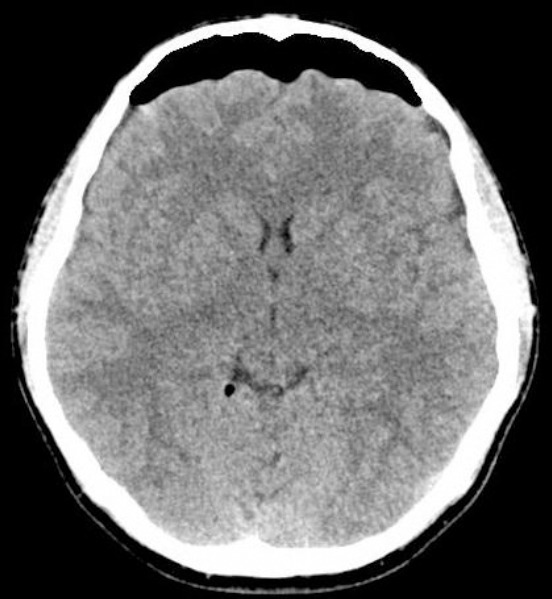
Symptomatic pneumocephalus in axial cranial CT obtained in postperative period*.
